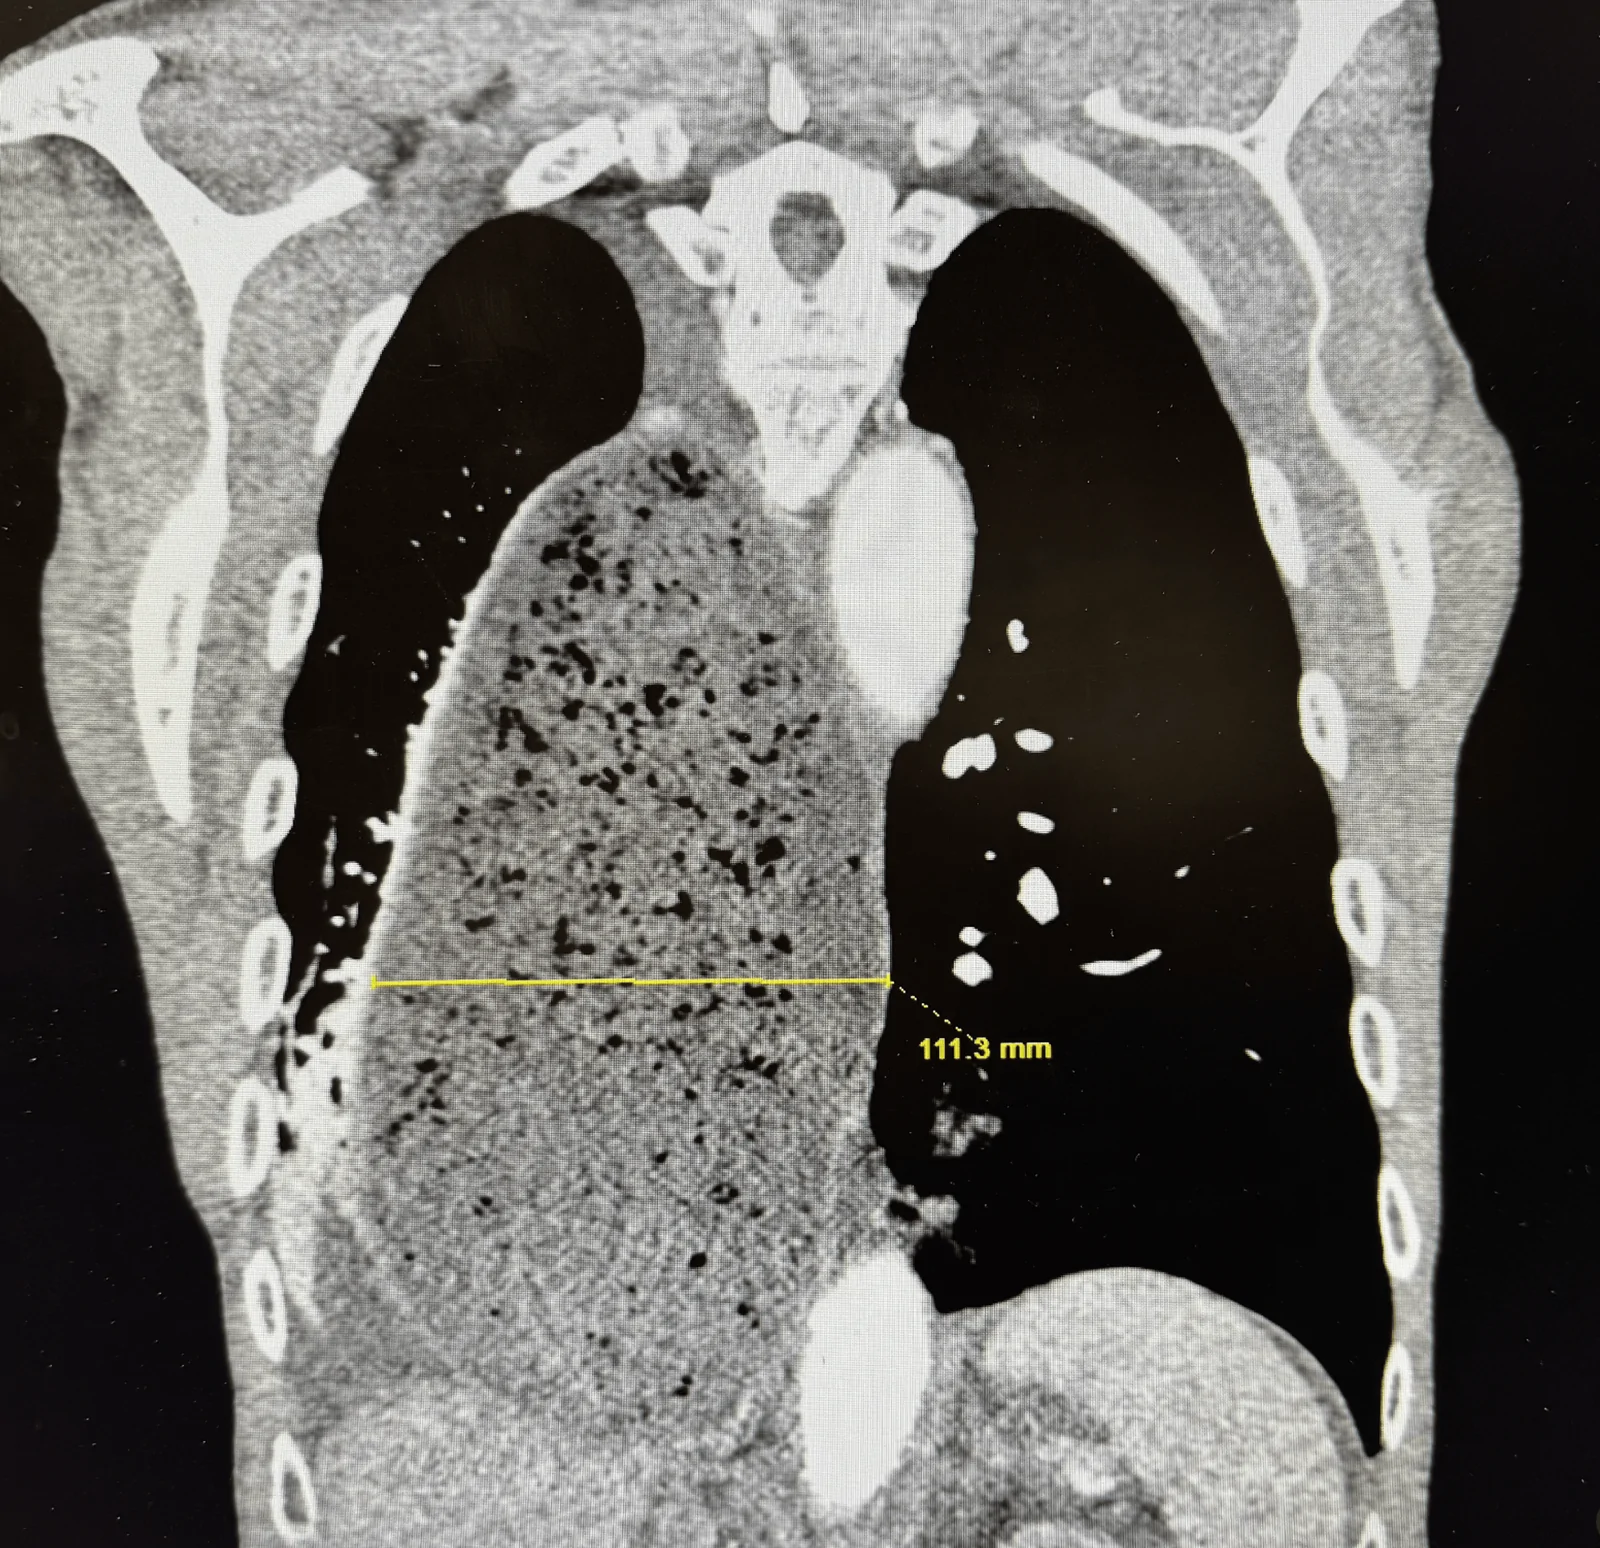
Coronal CT chest showing esophageal dilation (11 cm) with retained food debris
